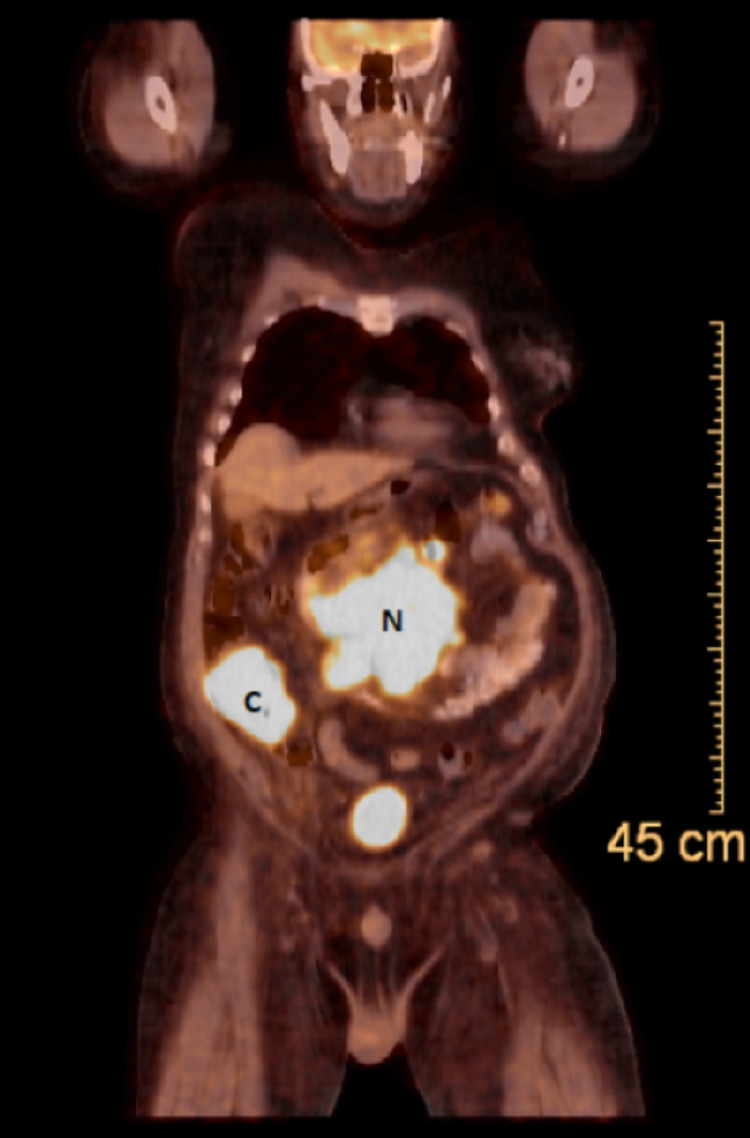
Positron emission tomography showing intense FDG uptake of the cecum (C) and retroperitoneal lymph nodal mass (N)
